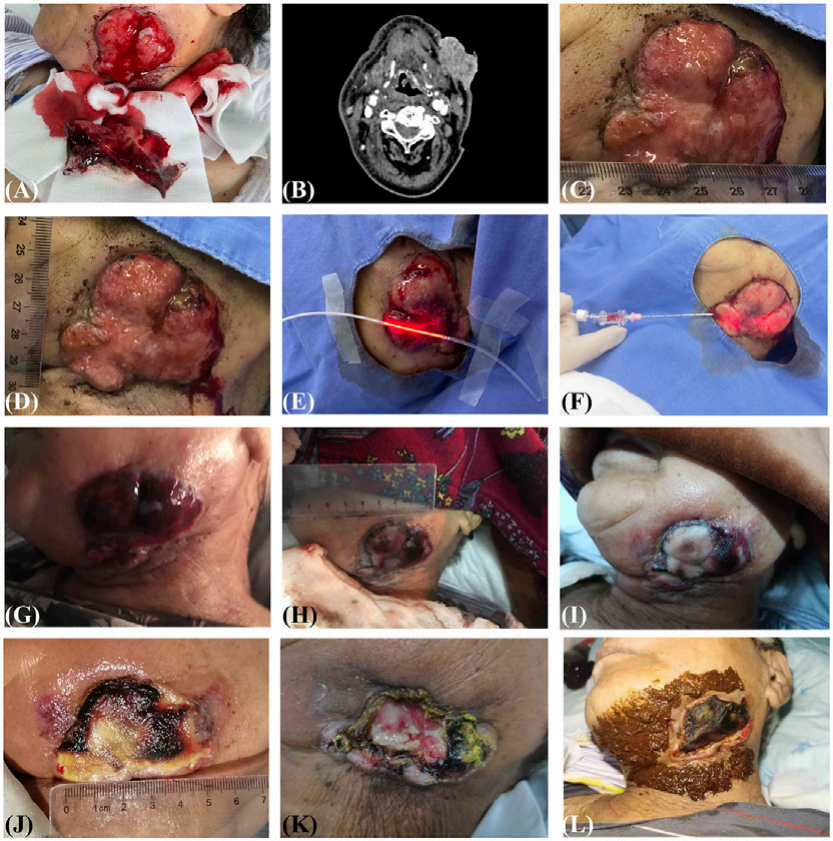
(A) A hemorrhagic red protruding mass on the left lower jaw (2021.09.28). (B) The enhanced neck CT scan indicated the left mandibular soft tissue tended to be malignant, with markedly uneven enhancement (2021.09.30). (C–F) The treatment process of PDT (2021.10.18). Measuring the size of pathological regions to decide specific surgical protocol (C,D), including superficial PDT (E) and interstitial PDT (F). (G–K) Postoperative changes of PDT. Acute local erythema, swelling, and pain were found around the tumor on the 2nd day (G). As time went by, the ulceration and hemorrhage of the left mandibular mass were gradually relieved (H, the 3rd day; I, the 5th day; J, the 8th day; K, the 41st day). (L) External use of CHM around the affected area with the Guwei method according to TCM theory. CHM, Chinese herbal medicine; CT, computerized tomography; PDT, photodynamic therapy; TCM, traditional Chinses medicine.
